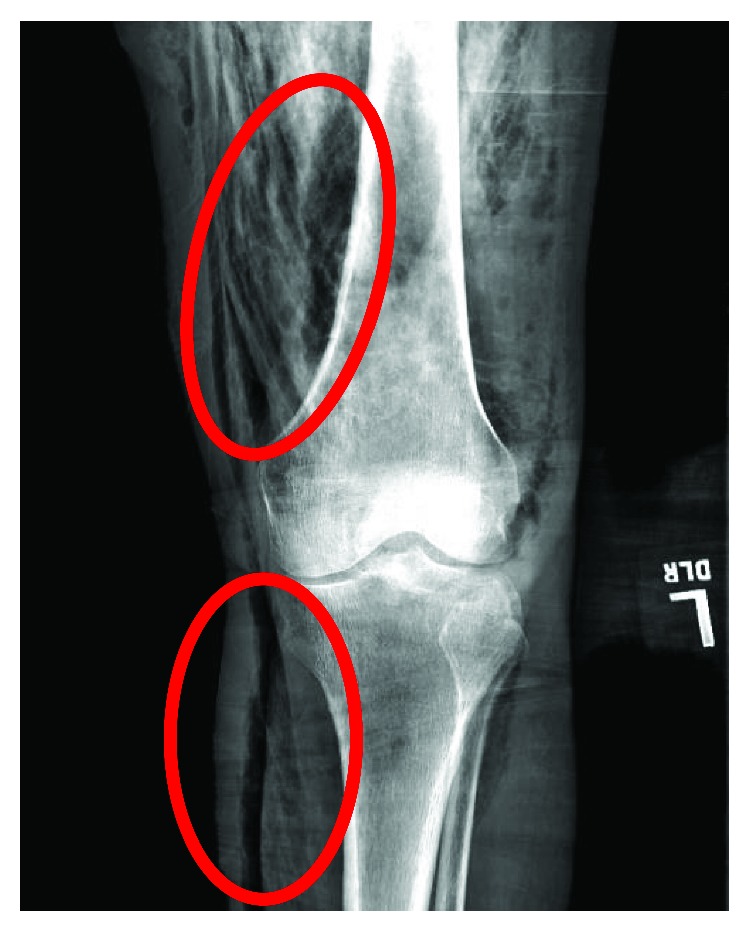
AP radiograph of the left lower extremity demonstrating subcutaneous emphysema (red ovals).
